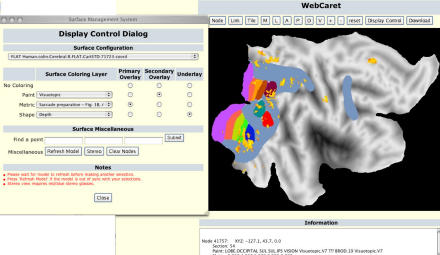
Using WebCaret and SumsDB to visualize functional magnetic resonance imaging activations and visual areas on a flat map of the human “Colin” cortical atlas (Image: David Van Essen; dataset with publication sources available: http://sumsdb.wustl.edu:8081/sums/directory.do?dir_id=702541)
